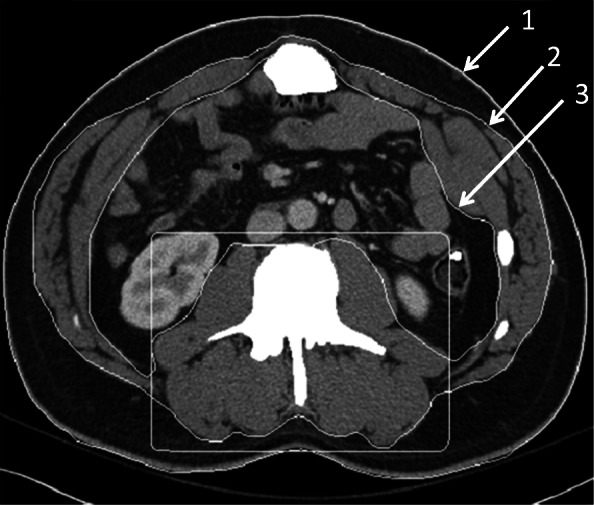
Example of segmentation of a computed tomography image with boundary lines between external air and subcutaneous fat (1), between subcutaneous fat and abdominal wall/paraspinal muscles (2), and between abdominal wall/paraspinal muscles and visceral fat (3). The box around the paraspinal muscles allows the algorithm to separately calculate the paraspinal muscle area (if this information is desired)
